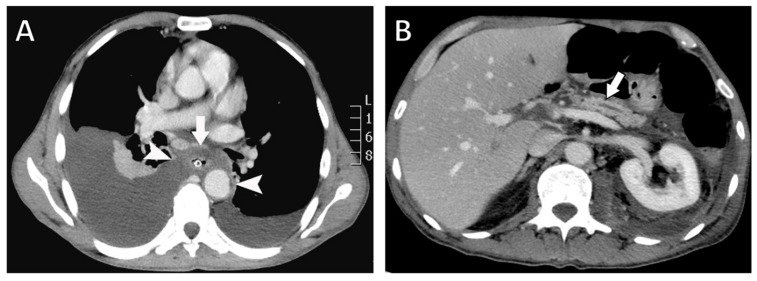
Contrast-enhanced computed tomography (CT) findings. (A) Axial CT image showing a fluid collection surrounding the esophagus (arrow) extending through the mediastinum and communicating with both pleural cavities (arrowheads). A nasogastric tube is visualized within the esophageal lumen. (B) Axial CT image demonstrating pancreatic atrophy with dilatation of the main pancreatic duct (arrow).
